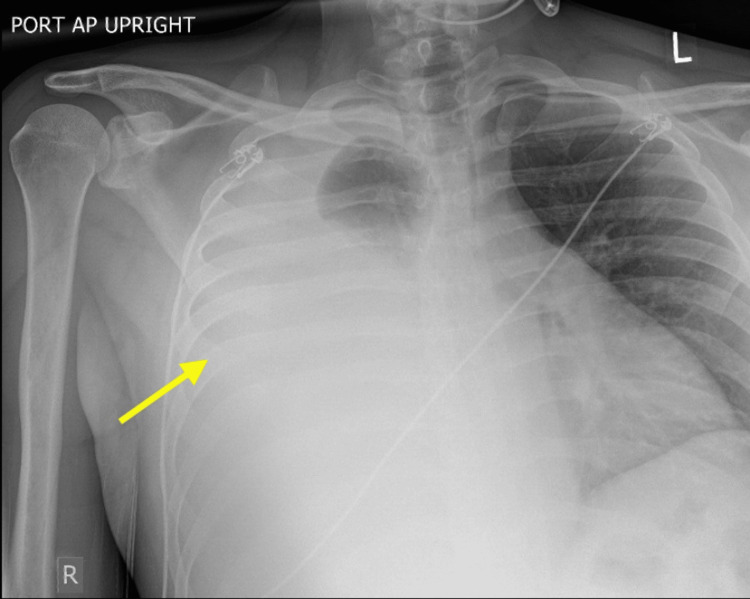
Initial chest X-ray The initial chest X-ray demonstrated opacification of the right hemithorax with minimal aeration of the right upper lung, reflecting a large pleural effusion (yellow arrow).
